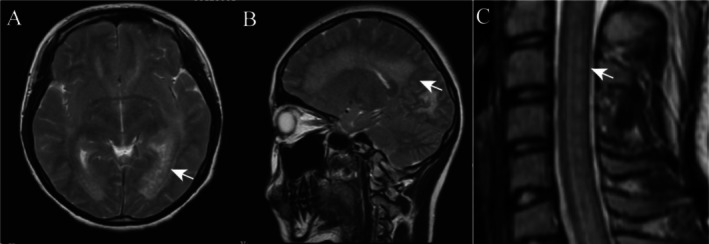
MRI brain w/wo before treatment, pre‐contrast. T2 weighted images (A–C) showed linear hypertensities adjacent to the fourth ventricle, bilateral lateral ventricles, cervical spine (indicated by arrows), and corona radiata and pons (not shown).
